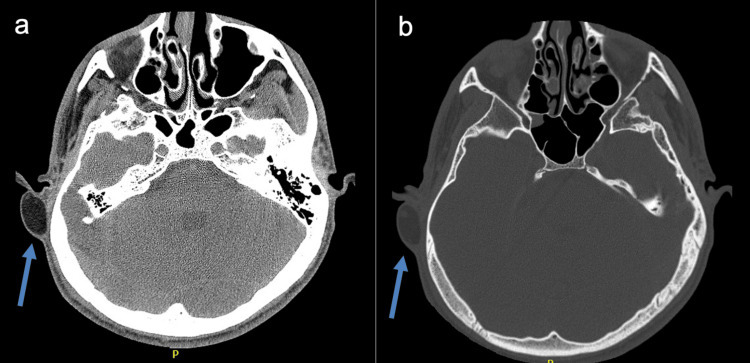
Axial computed tomography (CT) images of the temporal bone. (a) Soft-tissue window showing a well-circumscribed, low-attenuation cystic lesion in the right postauricular region, consistent with a dermoid cyst. (b) Bone window showing smooth cortical remodeling without evidence of bony erosion or intracranial extension. Blue arrows demarcate the location of the lesion.
